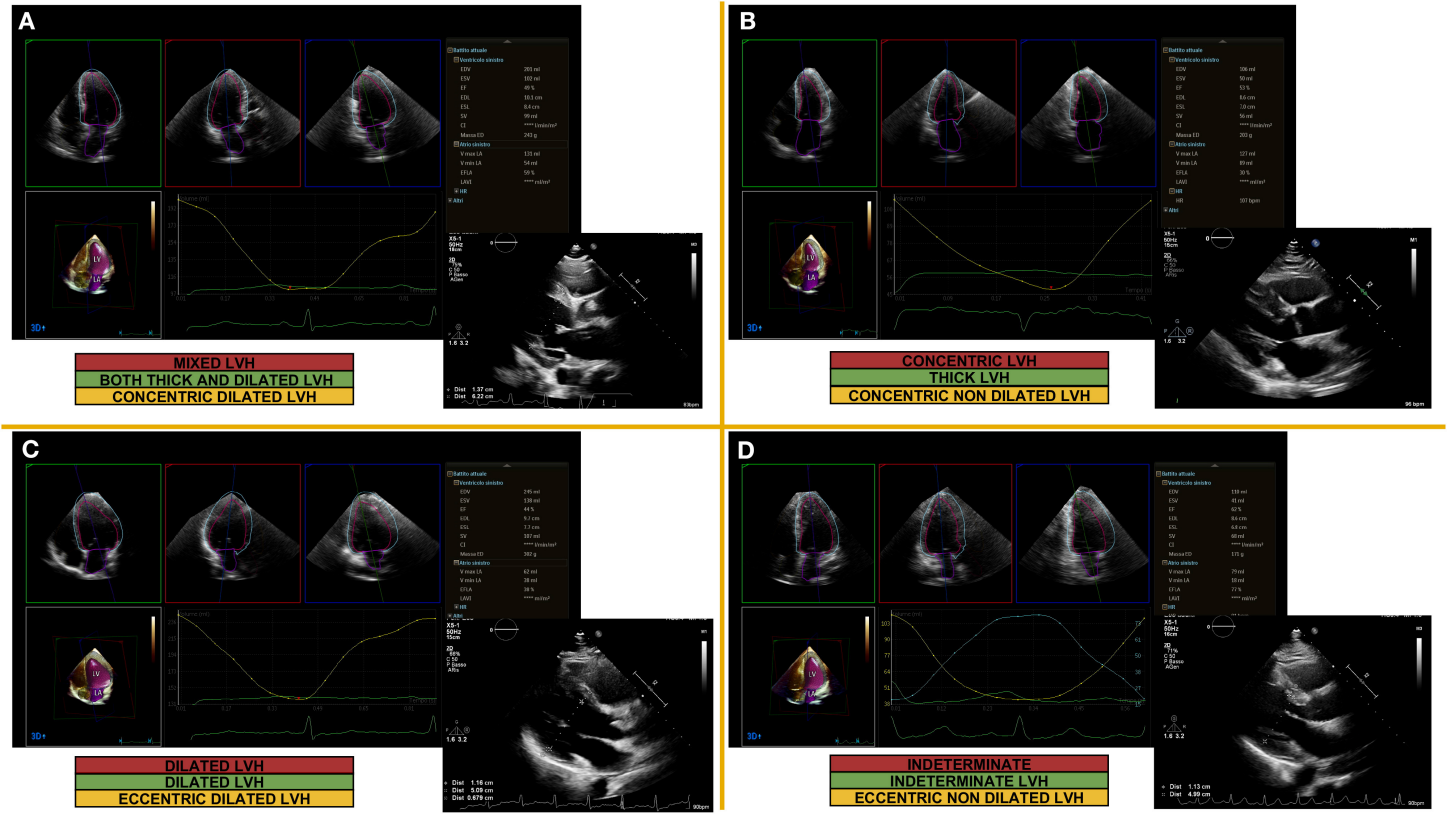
Examples of echocardiographic left lentricular hypertrophy classification based on concentricity, mass and volume quantification. Parasternal long axis view for linear 2D measurements (LV mass, concentricity) and 3D measurements (LV volumes) obtained from automated DHM (Dynamic Heart Model, Philips Healthcare, Andover, MA, USA) in a patient with mixed LVH (A), concentric LVH (B), dilated LVH (C), indeterminate LVH (D); 2D, two-dimensional; 3D, three-dimensional; LV, left ventricular; LVH, left ventricular hypertrophy.
